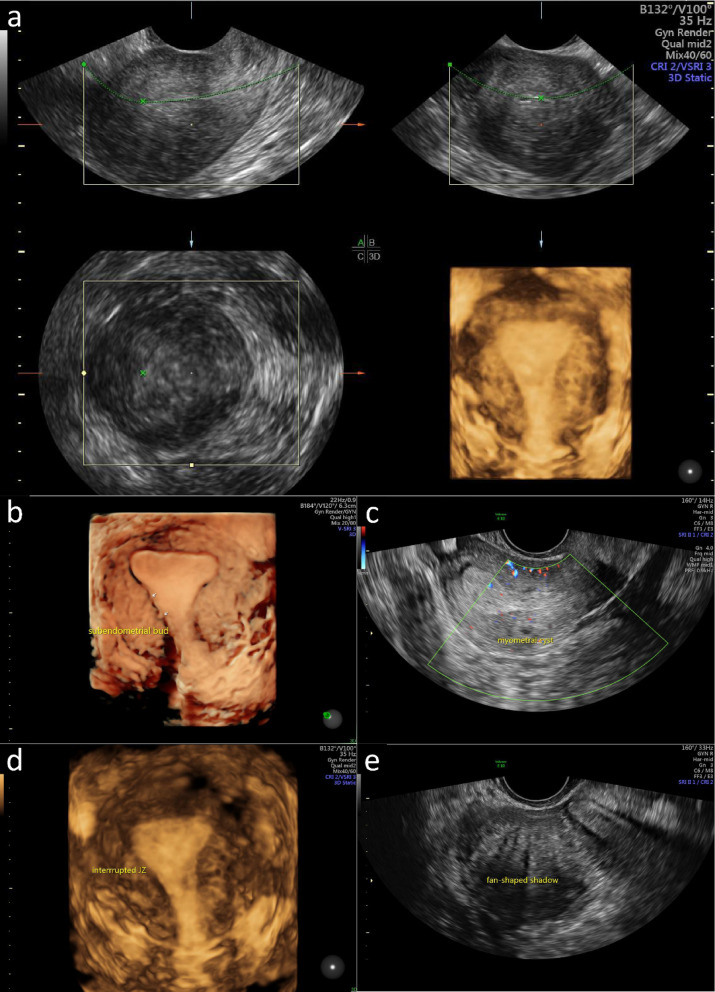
Transvaginal two-dimensional and three-dimensional ultrasound images of the patients in this study showing direct features (b, c) and indirect features (d, e) of adenomyosis as defined by the revised MUSA. (a) Acquisition of three-dimensional ultrasound images. (b) Subendometrial buds. (c) Myometrial cysts. (d) Interrupted junctional zone. (e) Fan-shaped shadowing.
